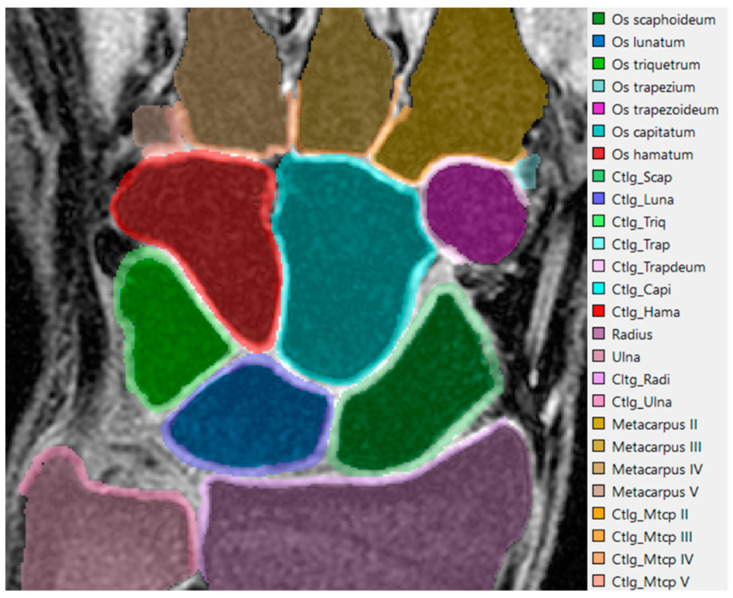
An exemplary segmented MR image slice of the wrist, the right side is the corresponding labels.
